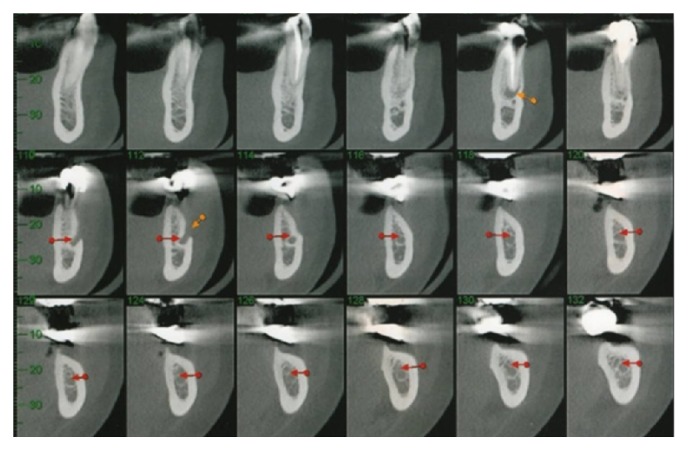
Computerized tomography (cone beam) left jaw.
